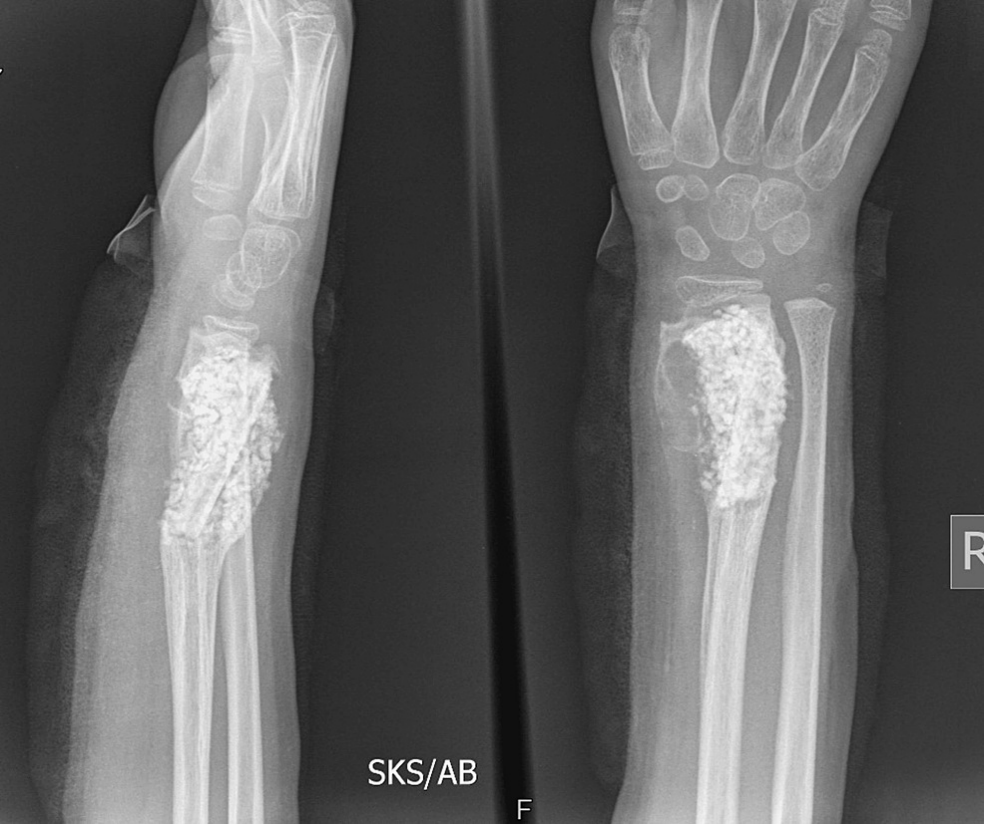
Check X-ray at the end of two months showing the incorporation of both fibular strut graft and synthetic bone graft
